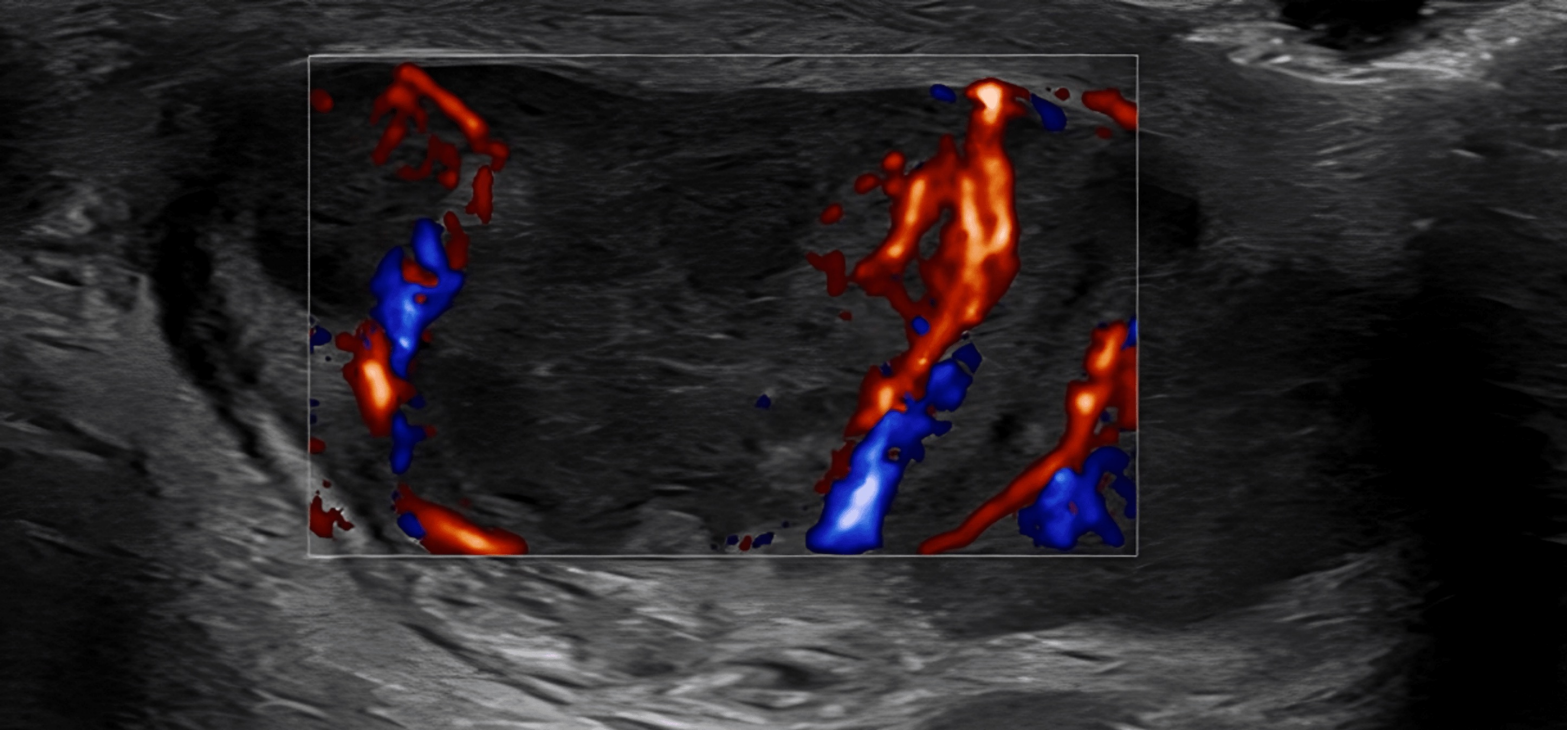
Ultrasound scrotum showing bilateral epididymo-orchitis.
